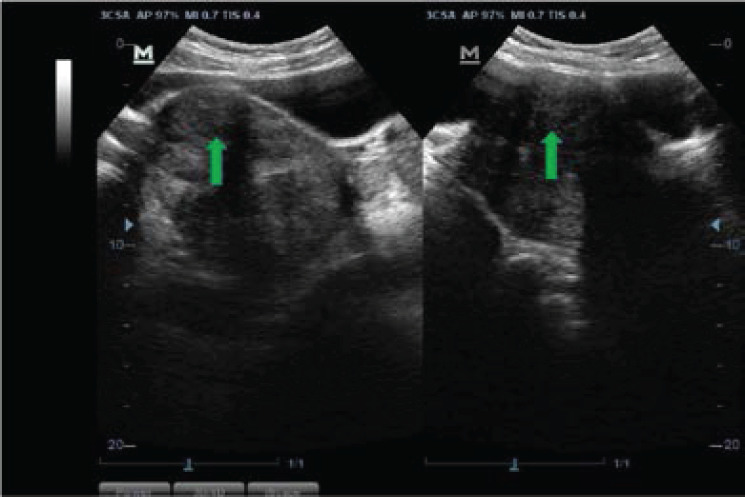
Ultrasound images of the uterus showing a myoma at the anterior uterine fundus (green arrows)
